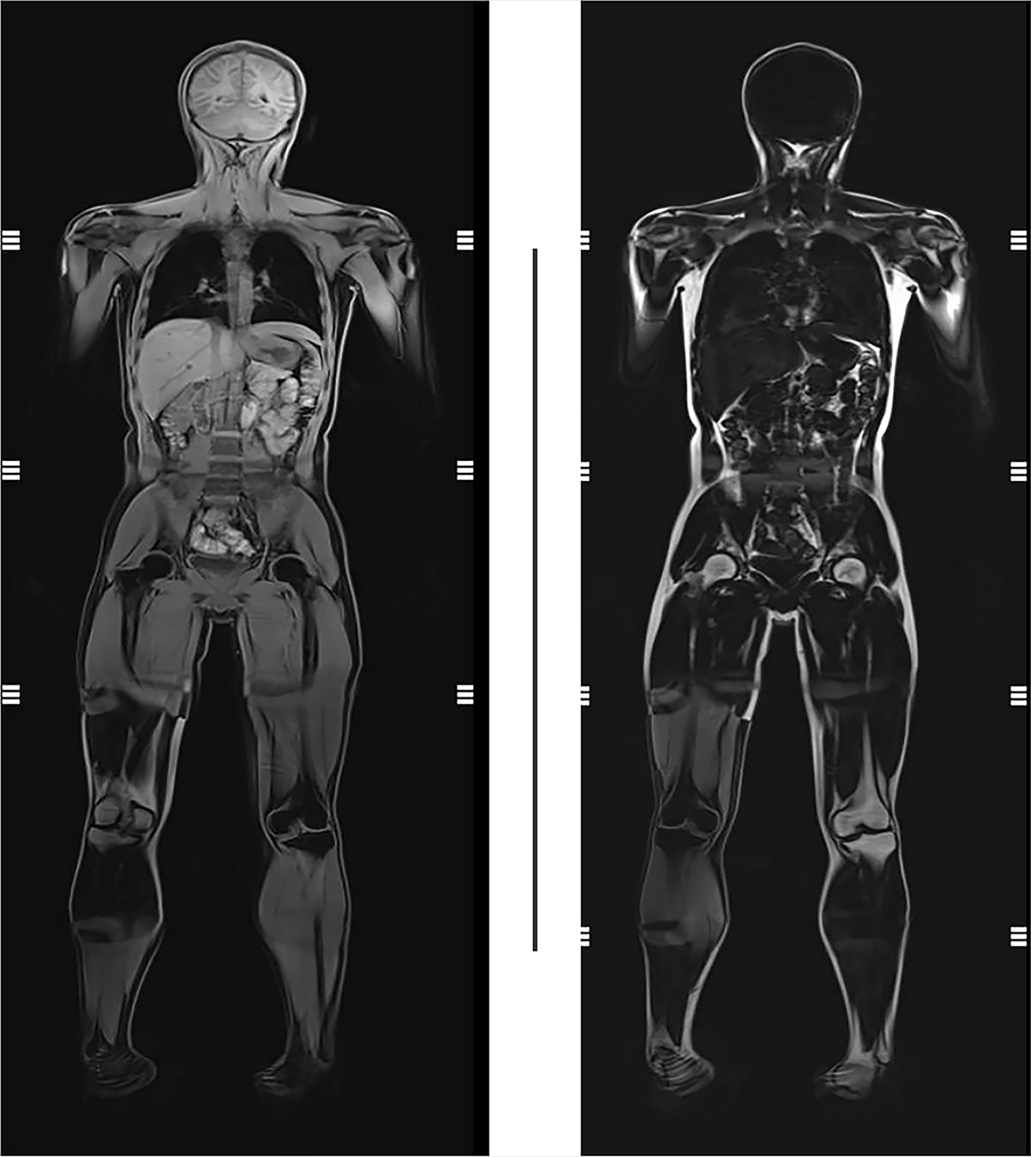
Quantitative MRI of fat of the proband. No reduction or abnormal distribution of fat was presented.
